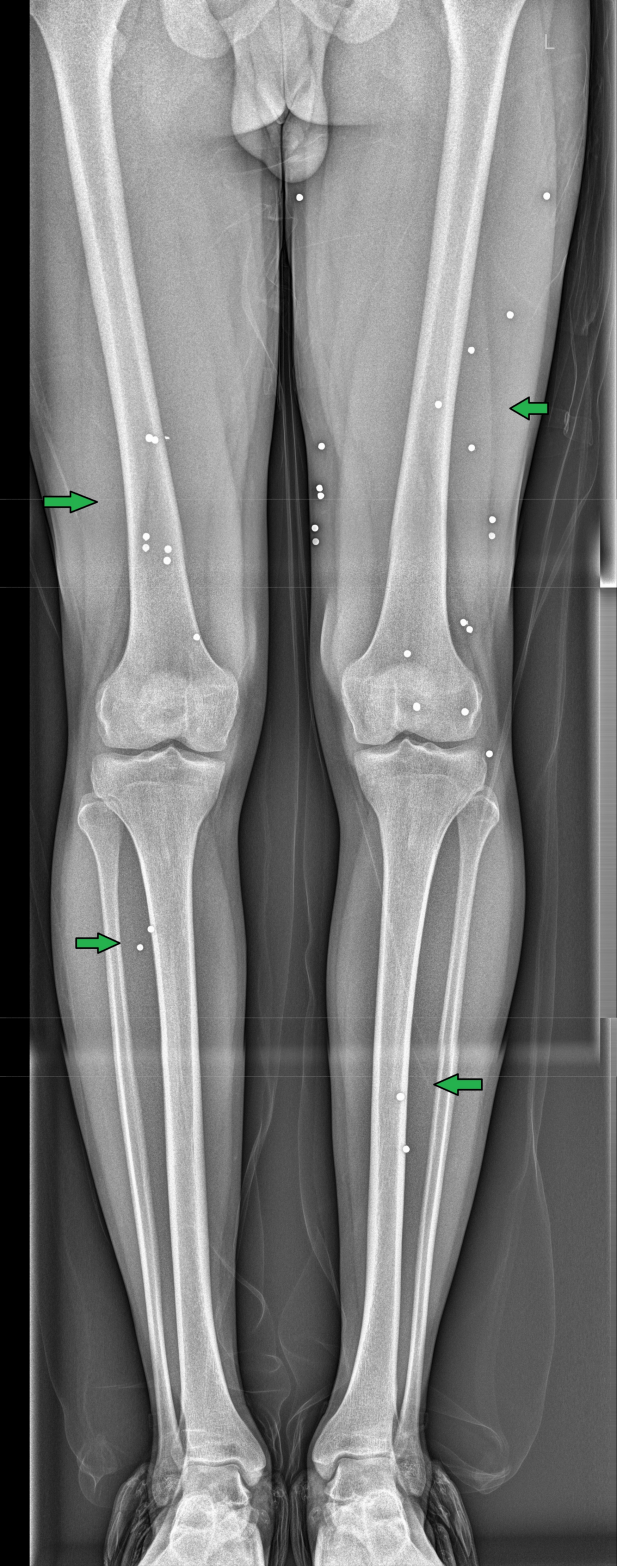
Full-length X-ray of both legs with the distal thigh and upper leg showing multiple well-defined radiodense foreign bodies which are reminiscent of tiny pellets. In comparison with the previous X-ray, there are no significant interval changes
